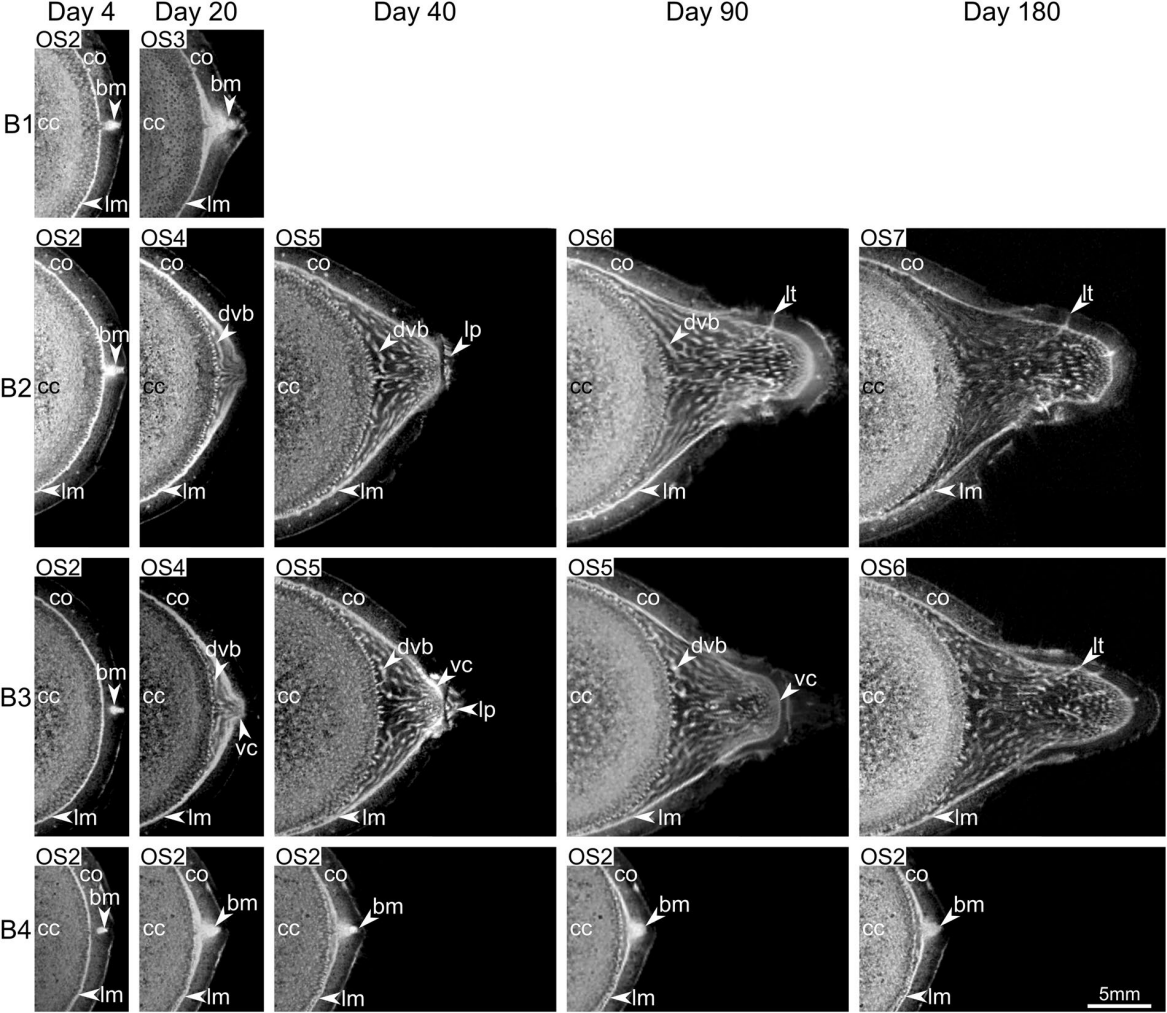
Axial MR images of the bud ontogeny in *Dracaena marginata*. The axial images are located in close proximity to the dotted line in Fig. 6. Rows: images of buds B1–B4 respectively. Columns: day 4-day 180 of image acquisition after decapitation. Bud B1 died back after 25 days and is therefore missing in the columns of day 40-day 180. The ontogenetic stage (OS2–OS7) for each individual image is given. bm: bud meristem; cc: central cylinder; co: cortex; dvb: developing vascular bundle; lm: lateral meristem; lp: leaf primordium; lt: leaf trace; vc: apical vegetative cone of the branch.
